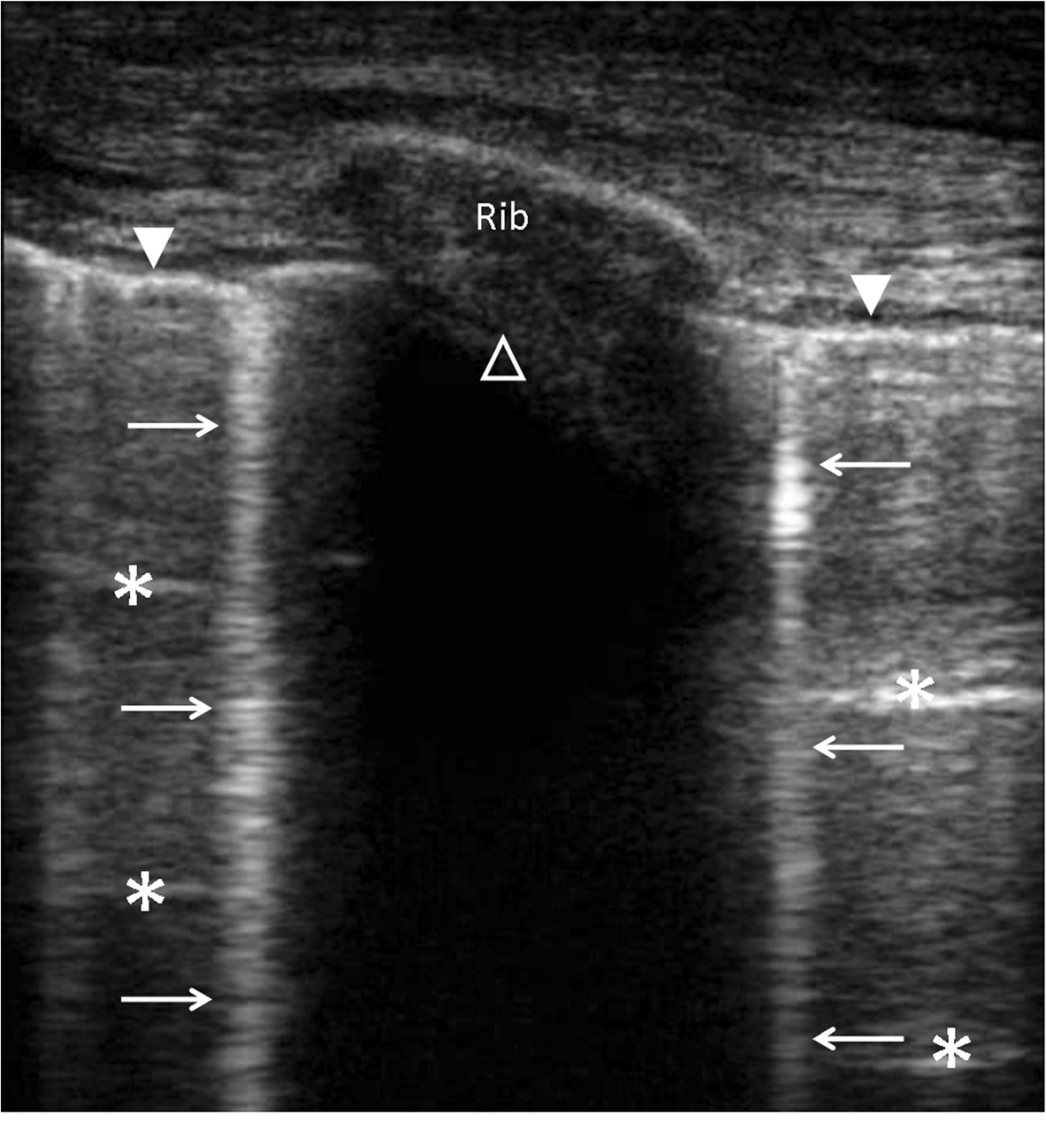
Longitudinal view of an intercostal space using chest ultrasonography disclosing the pleural line (*closed white arrow head*), A-lines (*white asterisks*), B-lines (*white arrows*), and shadowing related to the rib (*open white arrow head*)
